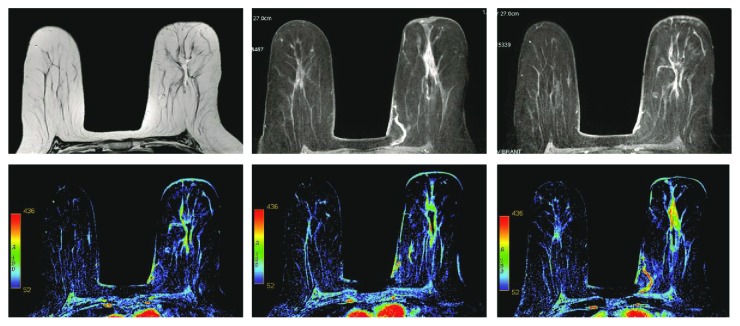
Breast MRI. Ill-defined deep retroareolar spiculate mass-like lesion extending over 3 × 1.5 cm revealing early enhancement peak with associated architectural distortion.
